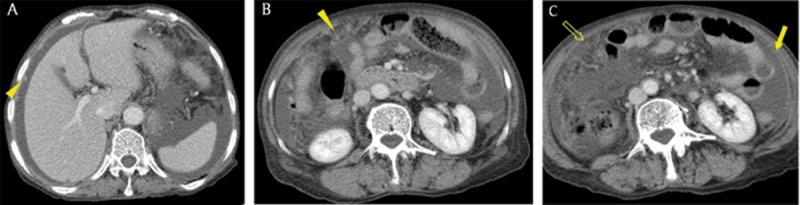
A 74-year-old woman with tuberculous peritonitis. Axial portal venous phase CT images showed multiple nodules (arrowheads), diffuse peritoneal thickening (arrow), ascites, and omental haziness (open arrow), findings that mimicked peritoneal carcinomatosis.
